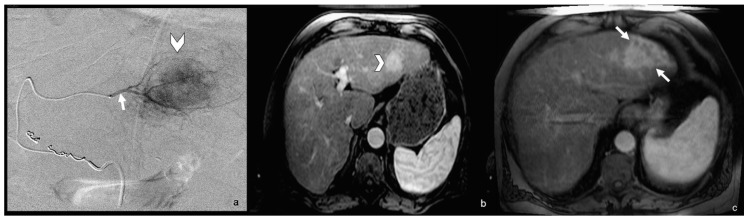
Radiation segmentectomy of HCC. (a) Angiogram of segmental tumor-supplying vessel (arrow) and tumor (arrowhead). (b) Pre-treatment MRI demonstrating arterial-enhancing tumor (arrowhead). (c) Post-treatment MRI showing radiation-induced changes in tumor-containing segment (arrows). Reproduced with permission from [43].
